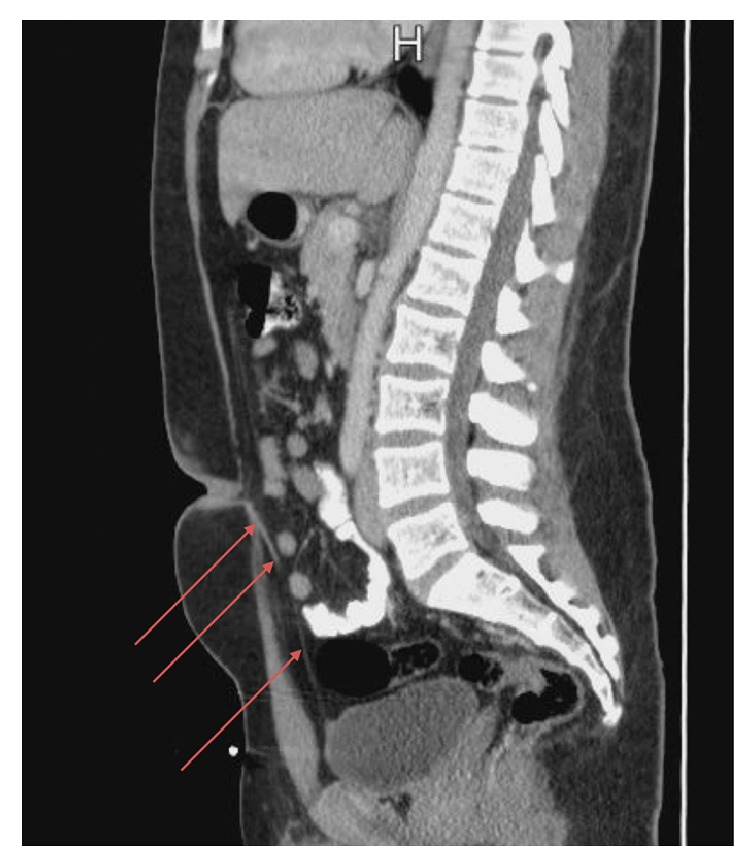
Computed tomography (CT) scan of the abdomen and pelvis sagittal plane showing a urachal remnant (red arrows) arising from the anterior/superior margin of the bladder and extending to the umbilical region. The remnant consists of a thin fibrous band of tissue measuring up to 4.6 mm in thickness near the umbilicus although measures as narrow as 2.0 millimeter along its course.
